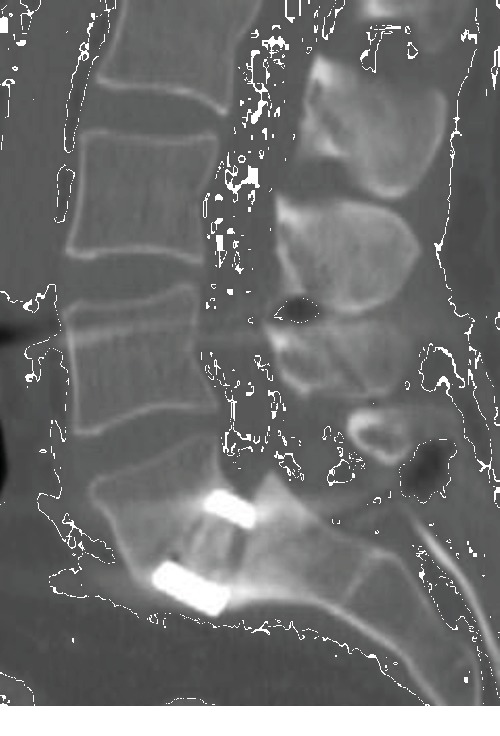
One-year CT scan control, highlighting the correct fusion of the L5-S1 level.
